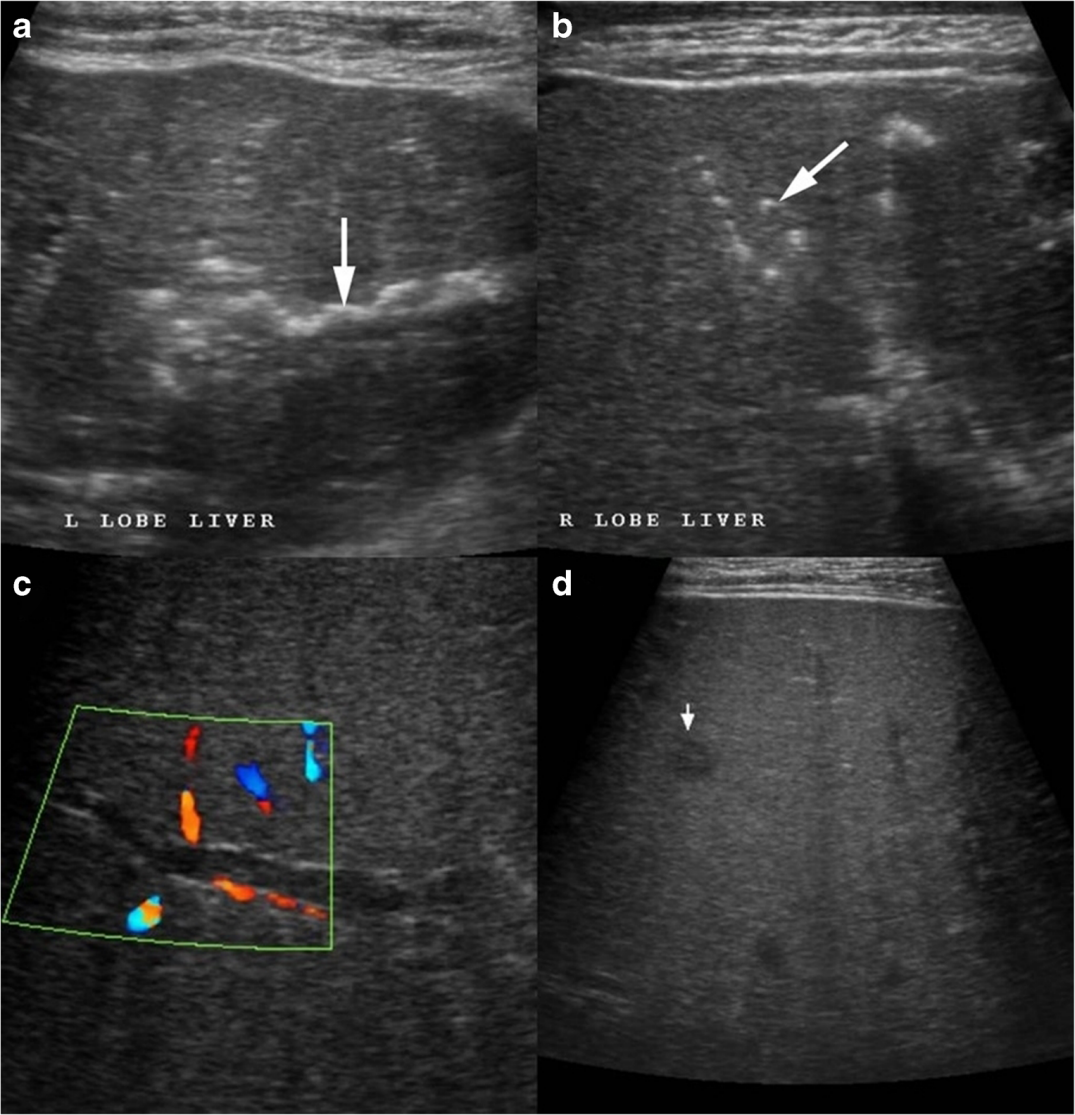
A 28-year-old man a known case of disseminated tuberculosis presenting with jaundice. a, b Ultrasound shows multiple areas of linear and miliary calcifications (*arrow*) in both lobes of liver. c Colour Doppler image shows dilated biliary radical with peripheral wall calcification. d Additionally, there is a well-defined small hypoechoic parenchymal lesion likely of a granuloma (*short arrow*)
